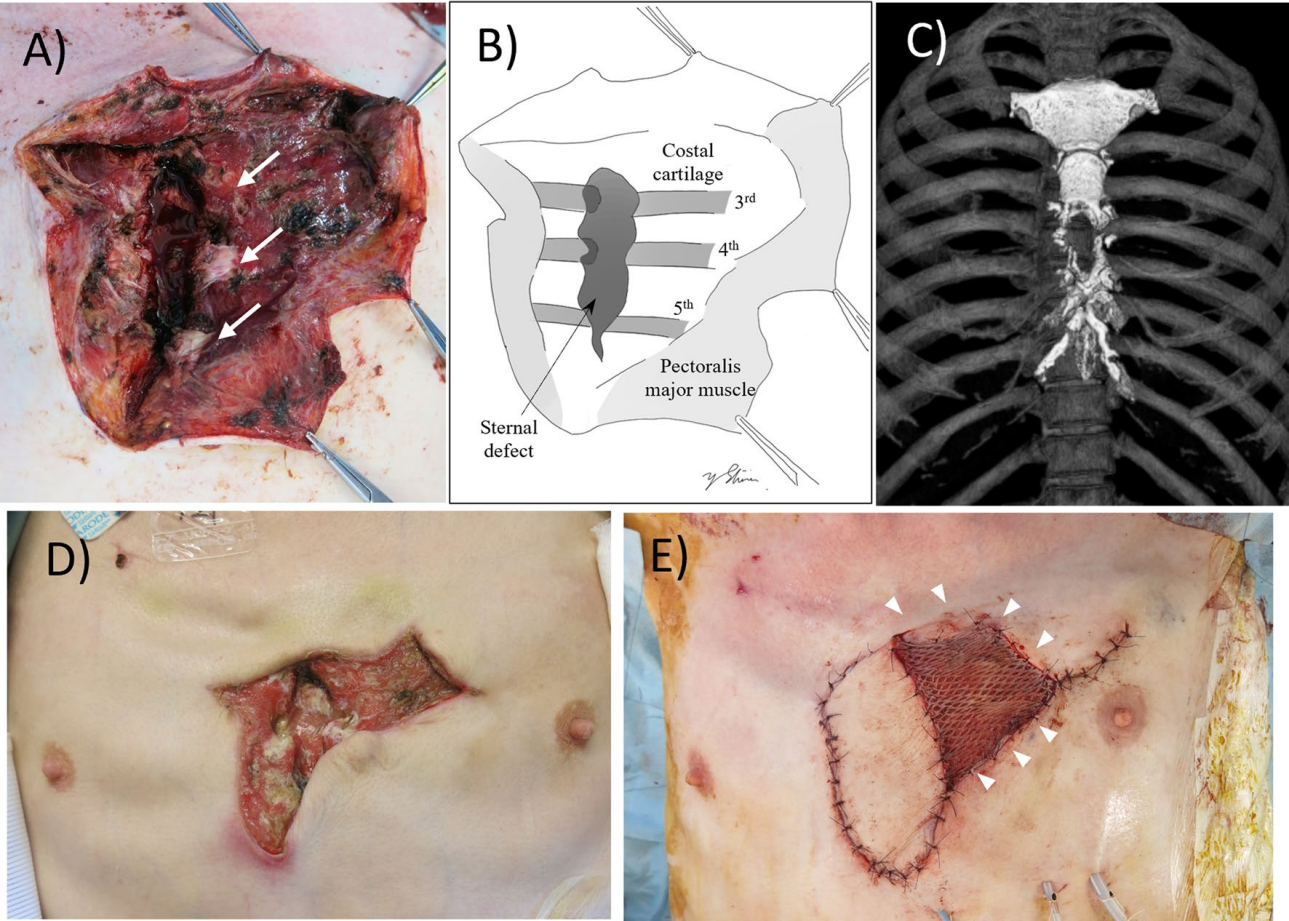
The sternal resection followed by latissimus dorsi myocutaneous flap reconstruction. A, B The anterior chest wall was widely incised, and the caudal half of the sternal body was resected leaving the costal cartilage attachments. The arrows show third, fourth, and fifth costal cartilage from the top. Light gray area shows remaining pectoralis major muscle, which was widely debrided. C Postoperative CT image showing sternal defect. D, E The anterior chest wall wound before (D) and after (E) latissimus dorsi myocutaneous flap reconstruction (arrows) combined with meshed split-skin graft (arrowheads)
